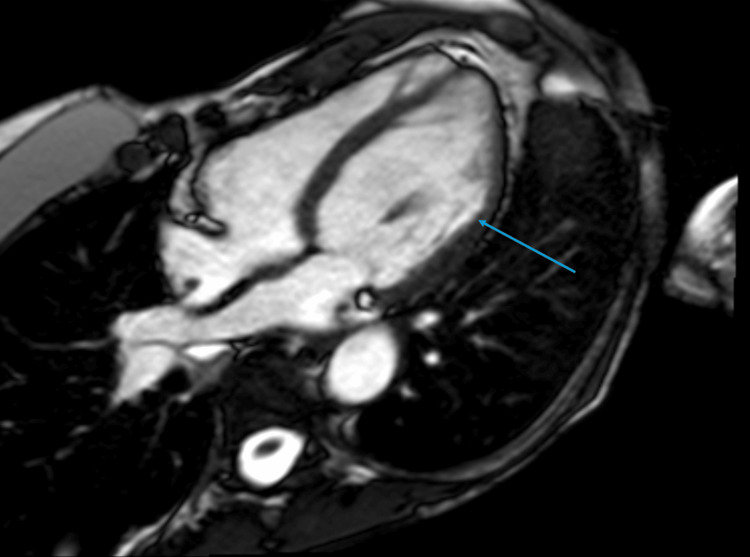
Cardiac MRI: apical four-chamber PSIR Blue arrow: Mid-myocardial, near transmural late gadolinium enhancement involving the mid-anterolateral and apical lateral wall segments. PSIR: Phase-sensitive inversion recovery
